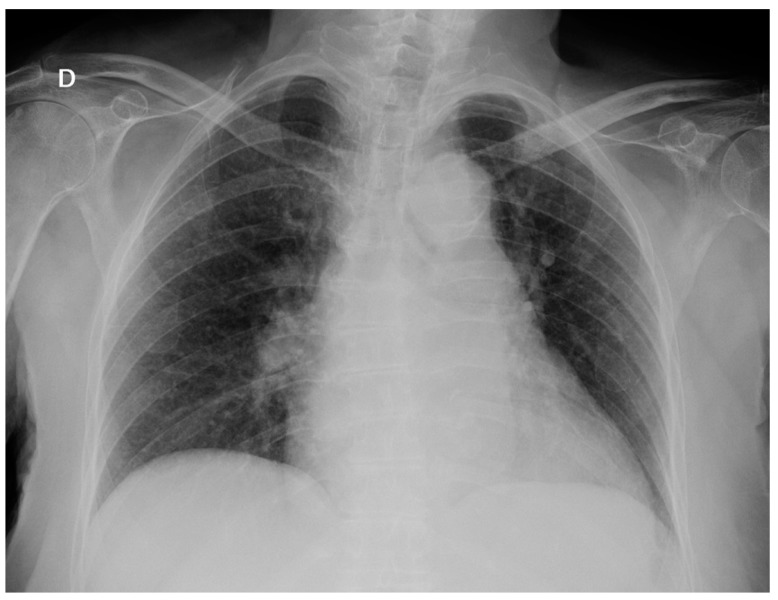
Chest X-ray on admission: there are no infiltrates or opacities.
